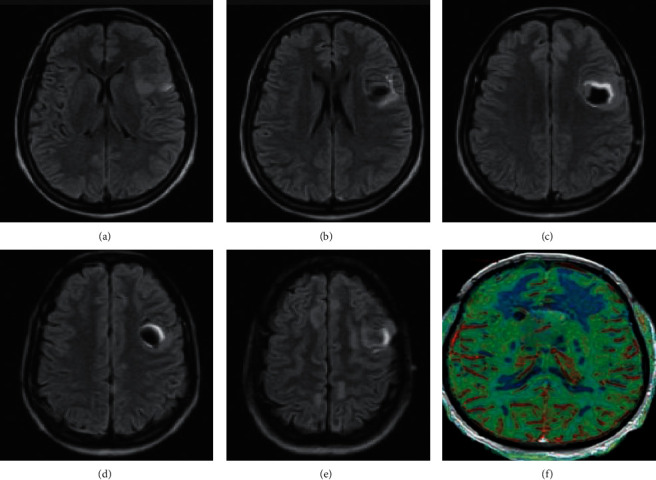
MRI images of primary intracranial melanoma. (A) (B), (C), and (D) : T1WI, T2WI, DWI, and PWI images of cerebral meninges region, respectively. (E) (F) : cervical spinal meninges region, respectively.
